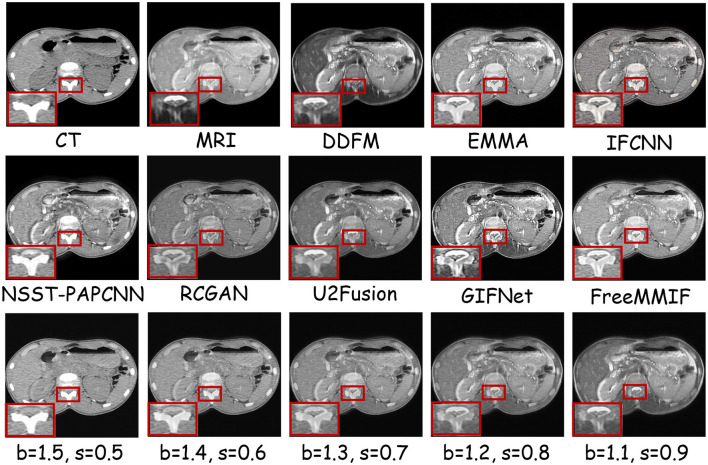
Visual comparison of CT-MRI fusion results.
